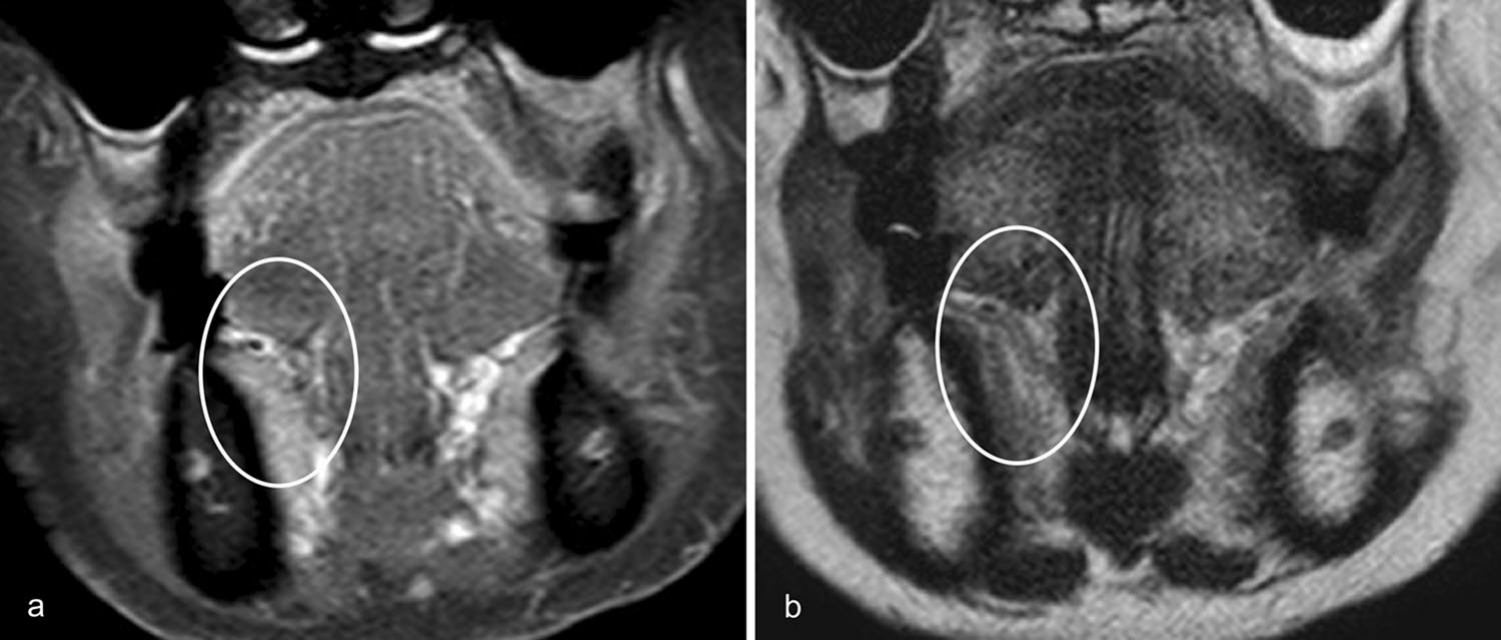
Undetectability of floor of the mouth cancer on MRI. An 88-year-old female with a right-sided squamous cell carcinoma of the floor of mouth. Both coronal fat-suppressed contrast-enhanced T1-weighted image (a) and coronal T2-weighted image (b) do not reveal any lesion (undetectable lesion) on the right floor of the mouth (within the circle) with clinically and pathologically proven right-sided squamous cell carcinoma of the floor of mouth
